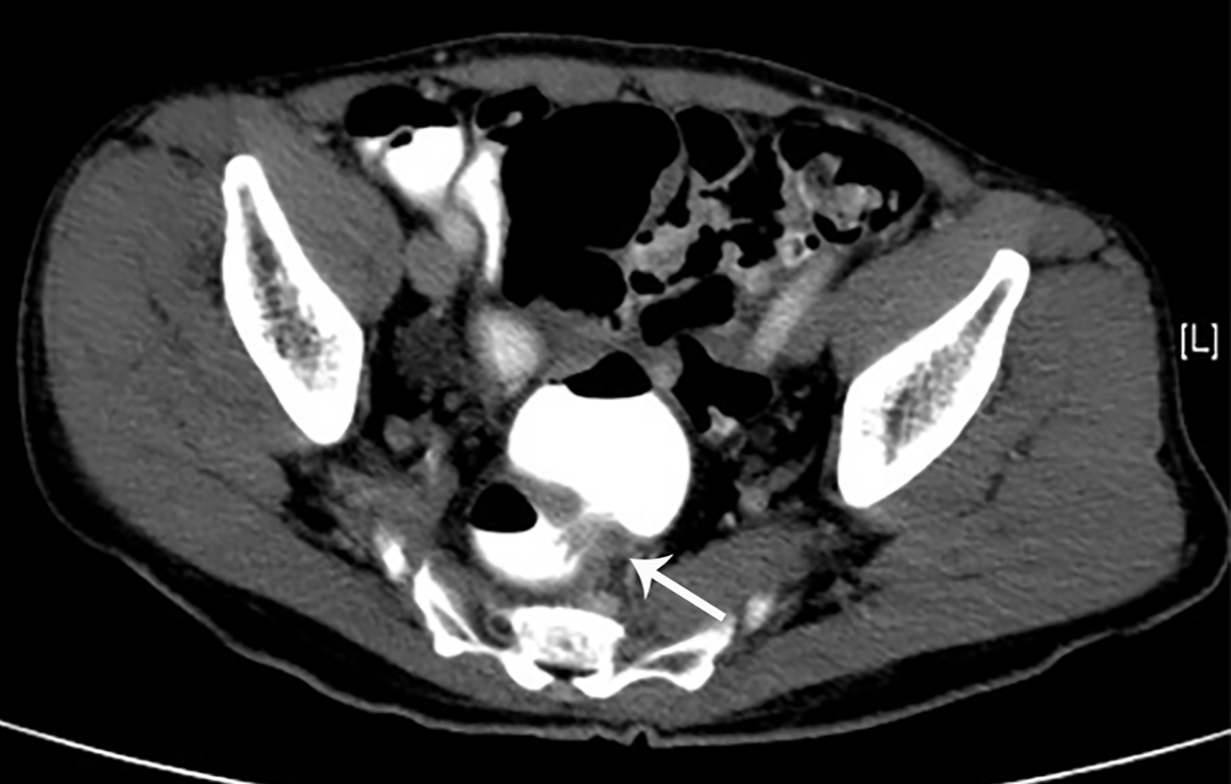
False positive category 2 scan–findings indeterminate for possible organic etiology and require further workup. Axial CT scan of a 74 year old male which demonstrates wall thickening of the rectosigmoid junction (arrow). Follow-up colonoscopy demonstrated no suspicious lesion at the rectosigmoid junction.
